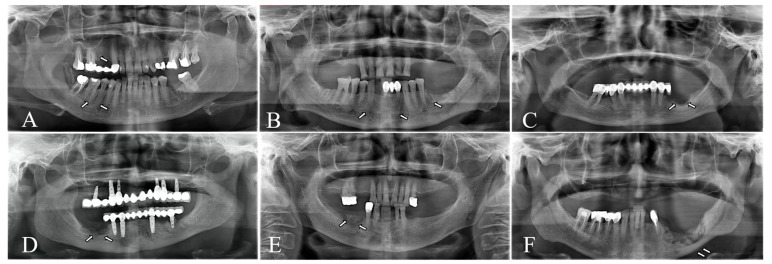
Radiographic parameters evaluated on digital panoramic radiographs. (A) lamina dura thickening, (B) trabecular bone alteration, (C) osteosclerosis, (D) cancellous bone destruction, (E) sequestrum formation, (F) periosteal new bone formation.
